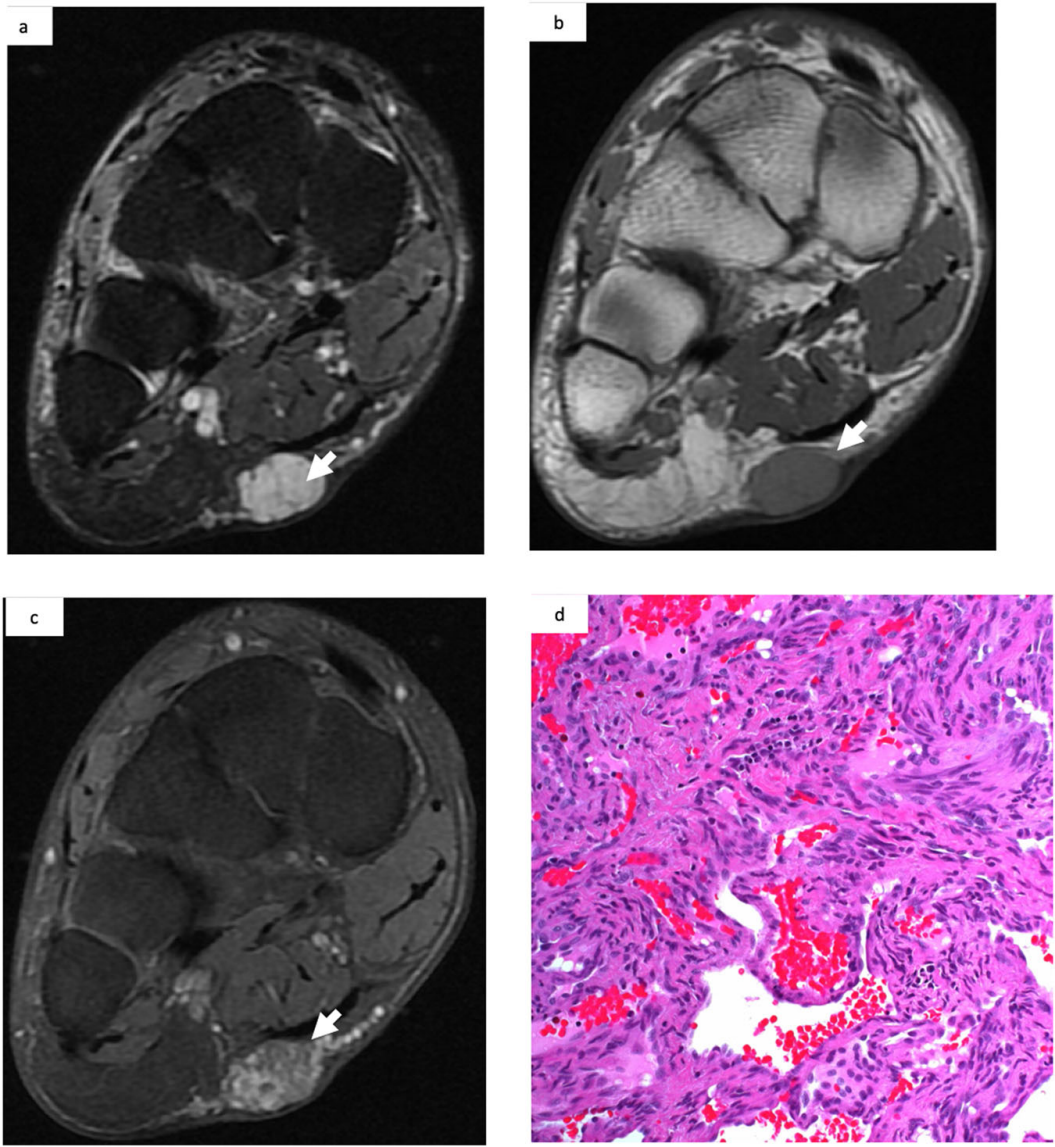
MRI of a 46-year-old woman with a painful mass on her left foot. a Hyperintensity appearance of the subcutaneous mass (white arrow) on T2WI with fat suppression. b Hypointensity appearance of the mass (white arrow) on T1WI. c Patchy enhancement (white arrow) on post-contrast T1WI with fat suppression. Absence of flow voids or presence of phleboliths on different sequences. d A spindle cell hemangioma confirmed by pathological analysis
